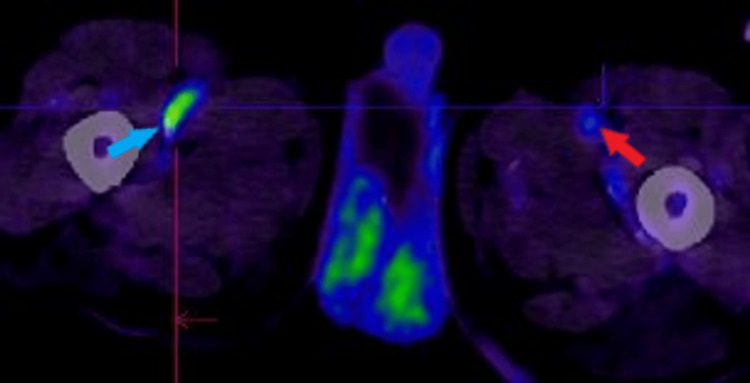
Fluorodeoxyglucose positron emission tomography (FDG-PET) scan demonstrating increased FDG uptake in the proximal right femoral artery, suggestive of active vascular inflammation.
